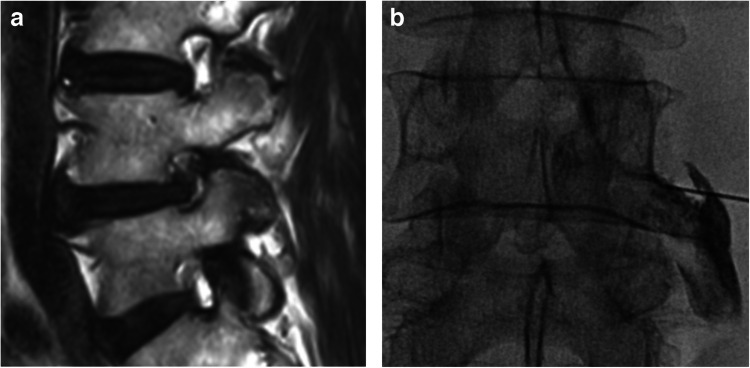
A 72-year-old male with diabetes mellitus presented with right leg pain started 10 years ago. a Lumbar spine MRI revealed severe foraminal stenosis at the right L4–L5 foraminal space, prompting a planned right L4–L5 transforaminal epidural steroid injection. b A fluoroscopy-guided right L4–L5 transforaminal epidural steroid injection was performed. The patient’s numerical rating scale score for pain was 5 before the injection and remained unchanged at 5 two weeks after the procedure
